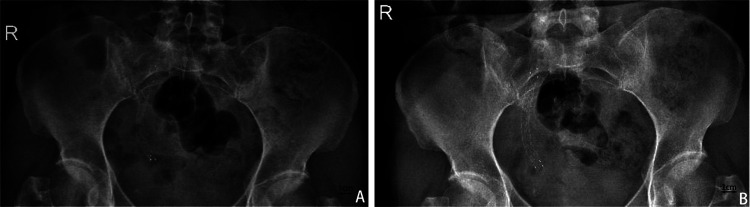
Abdominal X-ray to evaluate stent shape and position. (A) One month after the placement of Allium covered metal stent. (B) X-ray one year after the placement of Allium covered metal stent shows more complete stent release.
